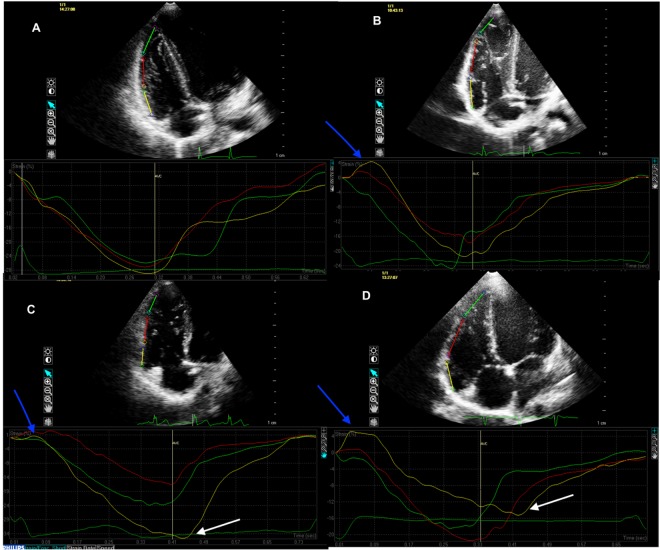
Speckle Tracking Echocardiography. Apical 4-chamber view. Postsystolic shortening. A. control, B study patient; prestretch present (+), PSS not present (-), C. study patient; prestretch (+), PSS(+) D. study patient; prestretch (+), PSS(+), deformation in BL lower than in ML. white arrow–postsystolic shortening, blue arrow–presystolic stretch, AVC–pulmonary valve closure.
